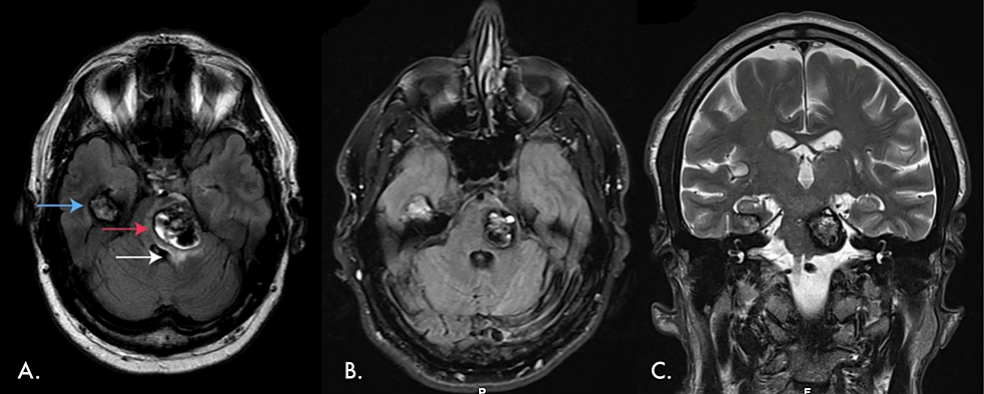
MR scan of the brain showing the pontine CM A: T2-weighted MR scan of the brain at presentation, showing the CM located in the pons (red arrow) and in the hyperacute bleeding phase with oedema (white arrow). The CM in the temporal lobe is also visible (blue arrow). B and C: T2-weighted MR scan of the brain, showing an axial and coronal view of the pontine CM in the non-bleeding phase. MR: magnetic resonance, CM: cavernous malformation
